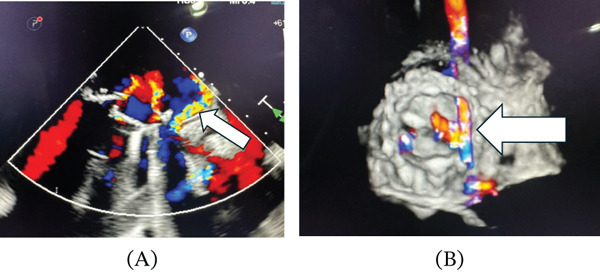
Two‐dimensional and three‐dimensional imaging with color Doppler. (A) TEE imaging at 105° omniplane. (B) 3D TEE imaging. The white arrows point to the PVL in each panel.
